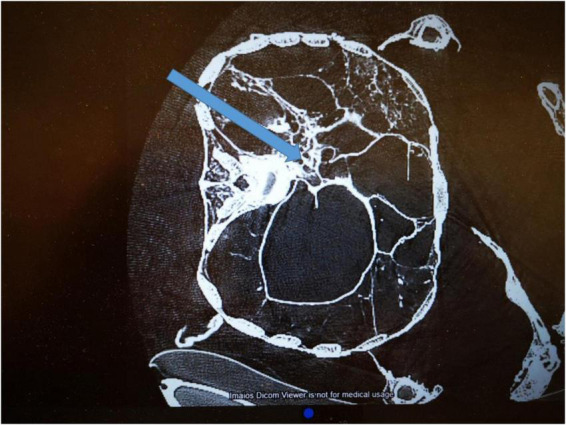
CT cross-section of the thorax at the level of the dorsal defect. The arrow indicates the presumed direction of an obvious stabbing wound reaching to the aorta and being surrounded by some major radiodense material.
